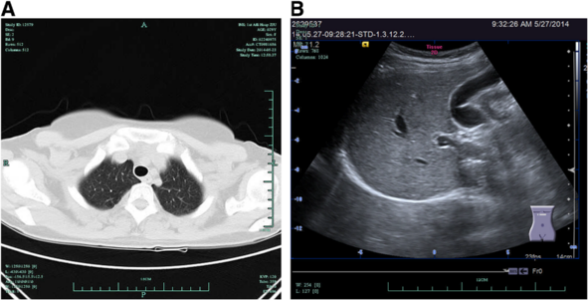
Lung imaging and follow-up examination. Lung CT scan (A) and abdominal ultrasonography (B) showed no obvious abnormality during the 1-year follow-up.
